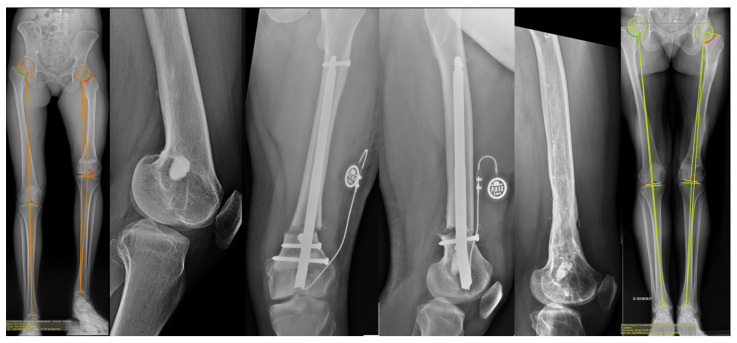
Radiographic images of a 26-year-old female with a 100 mm LLD associated with flexion and varus deformity due to growth plate injury at age 5 with subsequent attempted of Langenskiold procedure. 80 mm lengthening as well as correction in both the coronal and sagittal plane was performed with a retrograde FITBONE^®^ TAA nail.
